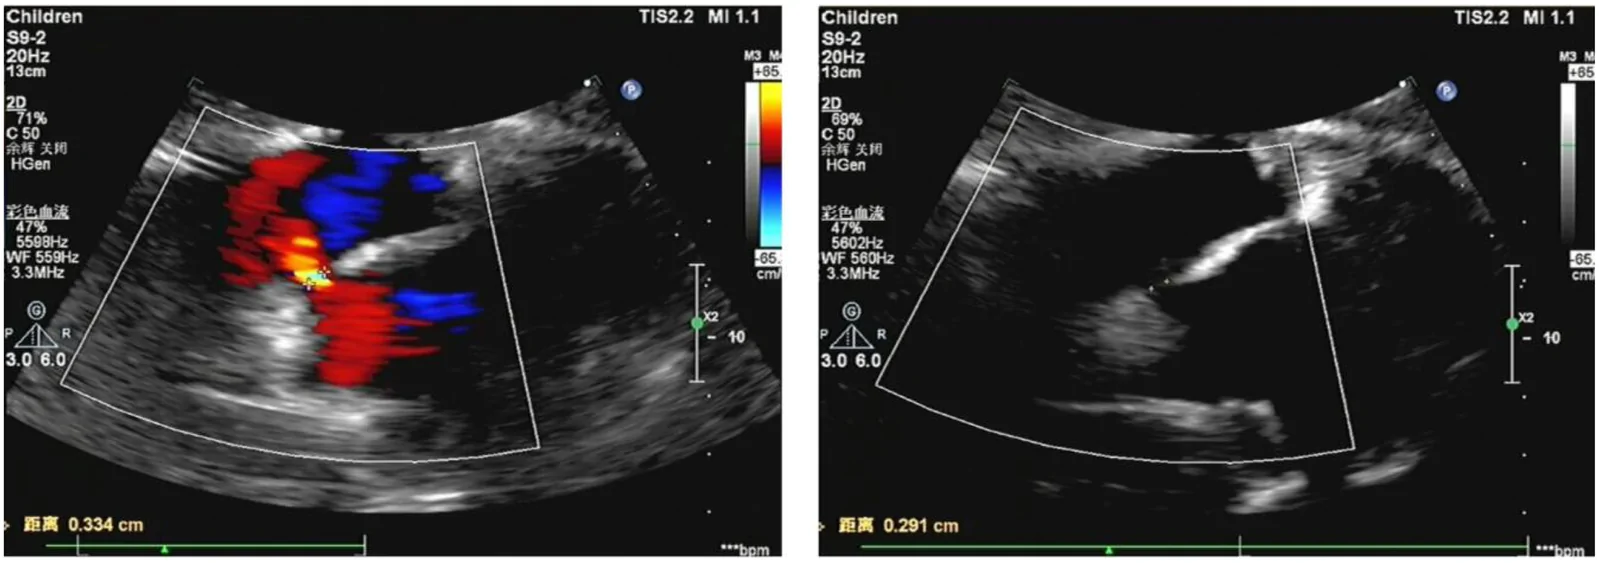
Echocardiographic image of the patient.
